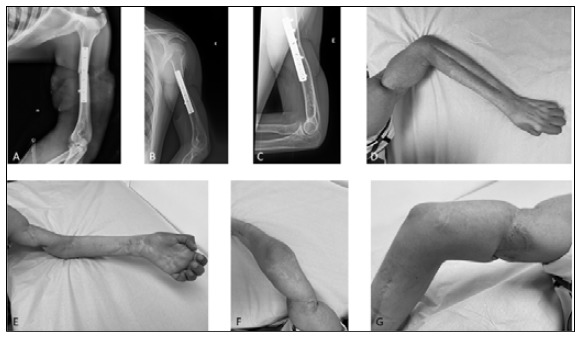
Case 5: (A) Postoperative radiography with synthesis with plate and screws; (B, C) X-ray after nine years of surgery, showing bone healing; (D, E, F, G) Clinical images of the upper limb after nine years.
